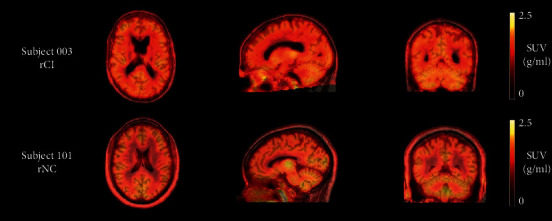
Orthogonal cross sections of coregistered PET and MR images from two representative patients (003 and 101). PET images are shown as SUV summed from 0–90 minutes. Images show regional heterogeneity consistent with the expected distribution of TSPO.
